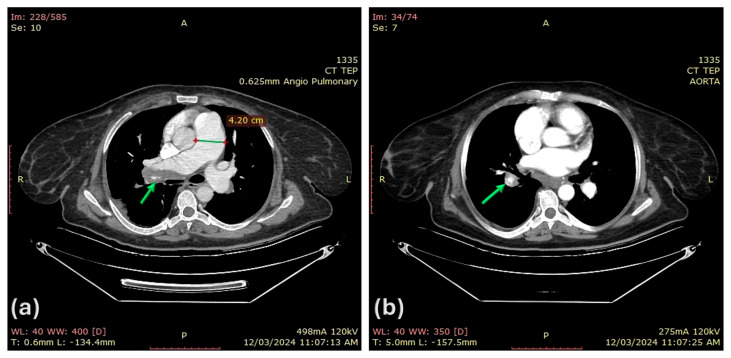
The chest CT angiography from 2024 reveals an enlarged pulmonary artery trunk and thrombosis (arrows) in the right pulmonary artery (a) and lower lobar pulmonary artery (b).
